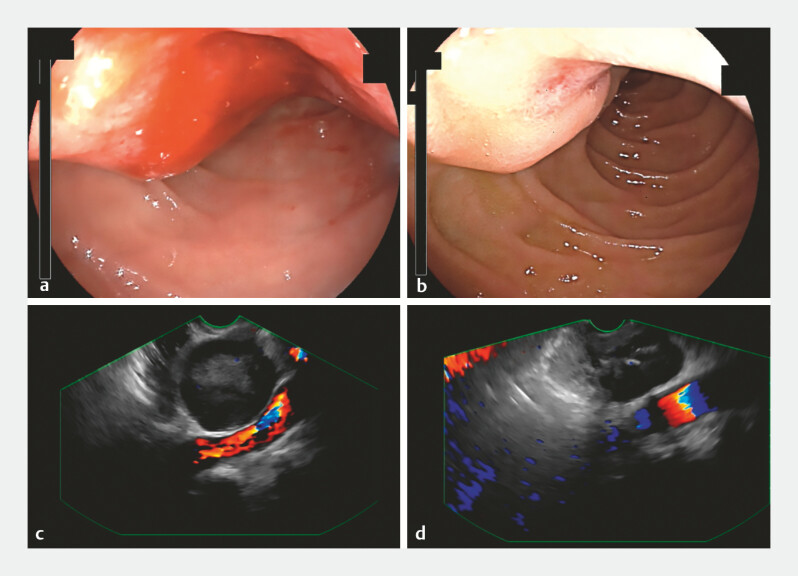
Gastroscopy and endoscopic ultrasound (EUS) images.
